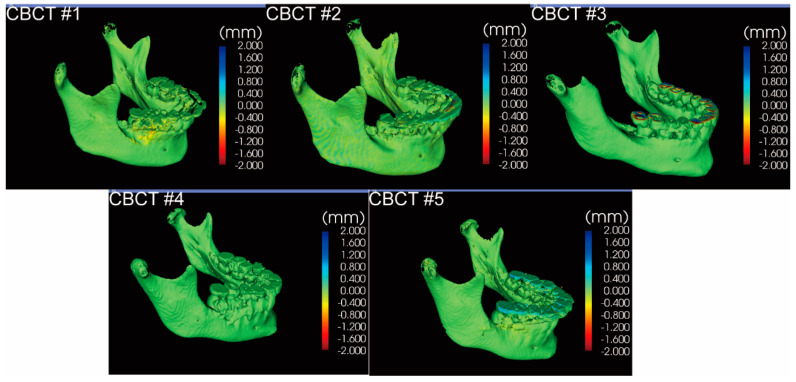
The model-to-model distance analysis in the 3D Slicer software for two segmentations performed by one examiner for each CBCT.
